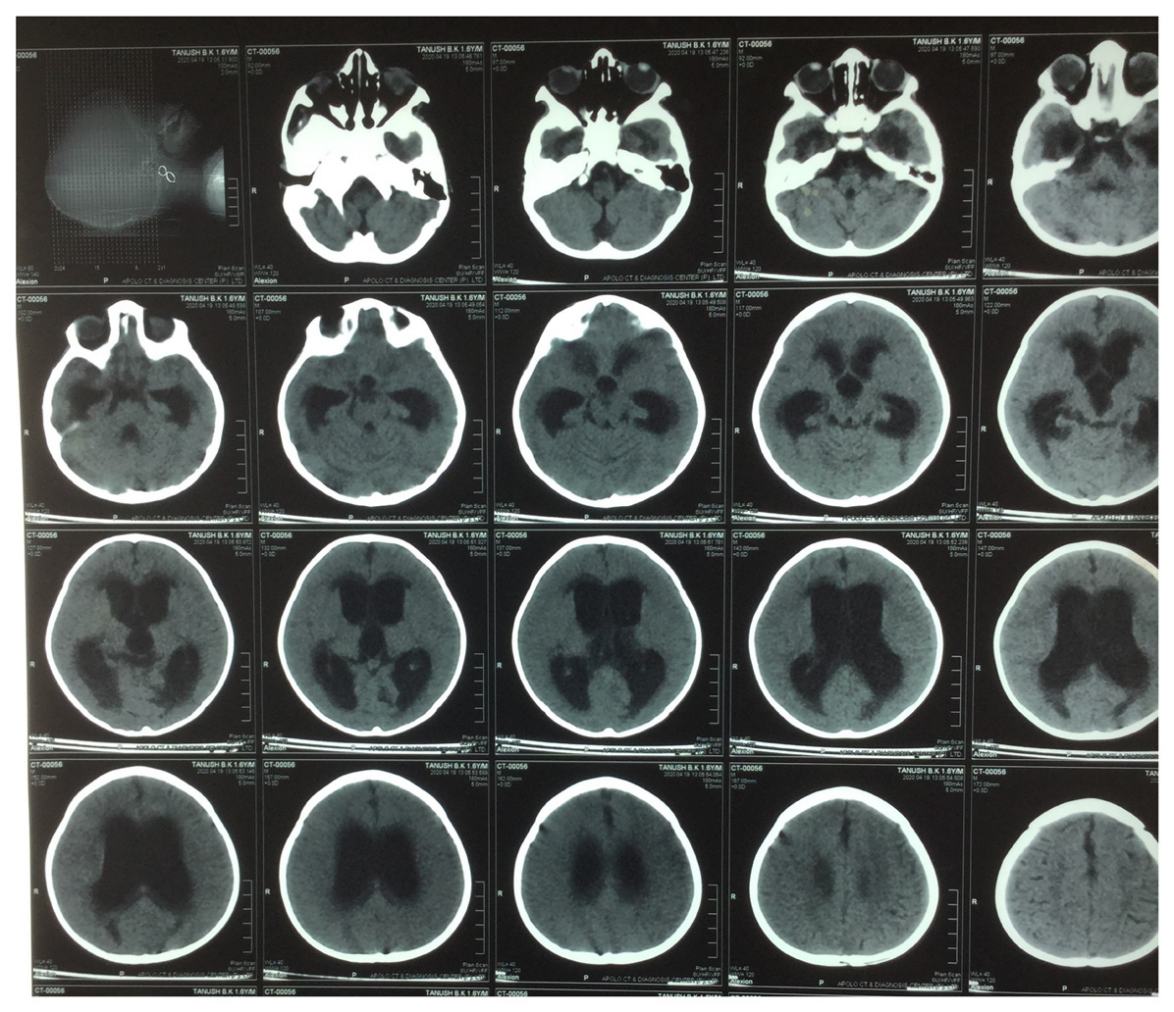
Plain computed tomography (CT) of the brain showed moderately dilated bilateral lateral and third ventricles with non-dilated fourth ventricle and periventricular oozing in the bilateral frontal, temporal, and occipital horn, suggestive of non-communicating hydrocephalus.
